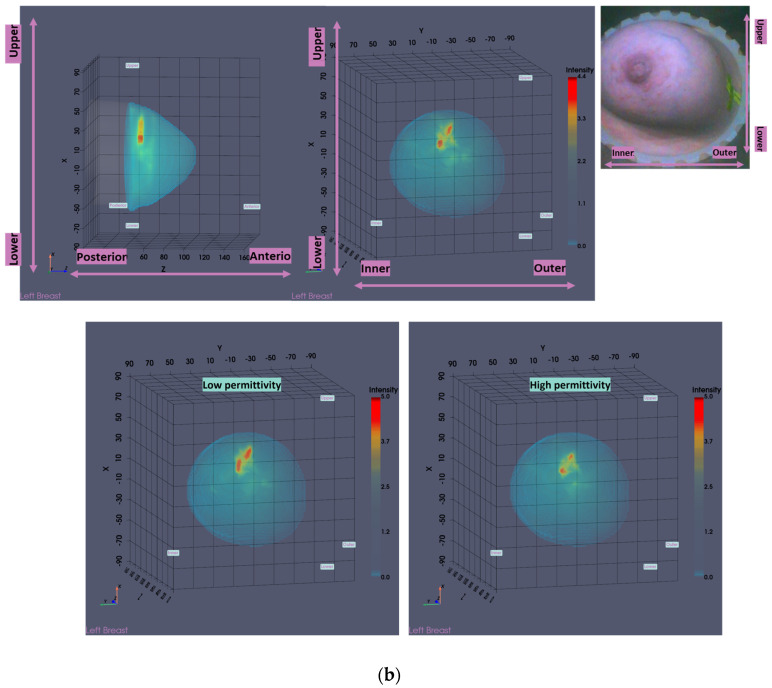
P003-L—Invasive Ductal Carcinoma: Layout of the imaging dataset: (a) Reference patient study data. Top row: annotated CC and MLO mammographic views of the left breast performed after the biopsy of the IDC lesion and a snapshot of the annotated ultrasound scan of the breast provided as reference data for the same lesion in this study. Bottom row: Spatial maps of the dense tissue thickness, provided as customized output of Volpara Lab on the CC and MLO mammographic planes, and Volpara Lab computational outputs to define Volumetric Breast Density (VBD). (b) Wavelia imaging outputs. Top row: Wavelia MWBI Global Averaged 3D volumetric image (Default View and Sagittal View) and endoscopic camera view of the breast after positioning in the MWBI scanner—the depicted view is aligned with the orientation of the MWBI 3D image’s Default View. Bottom row: Wavelia MWBI low-permittivity and high-permittivity 3D volumetric images presented in Default View.
